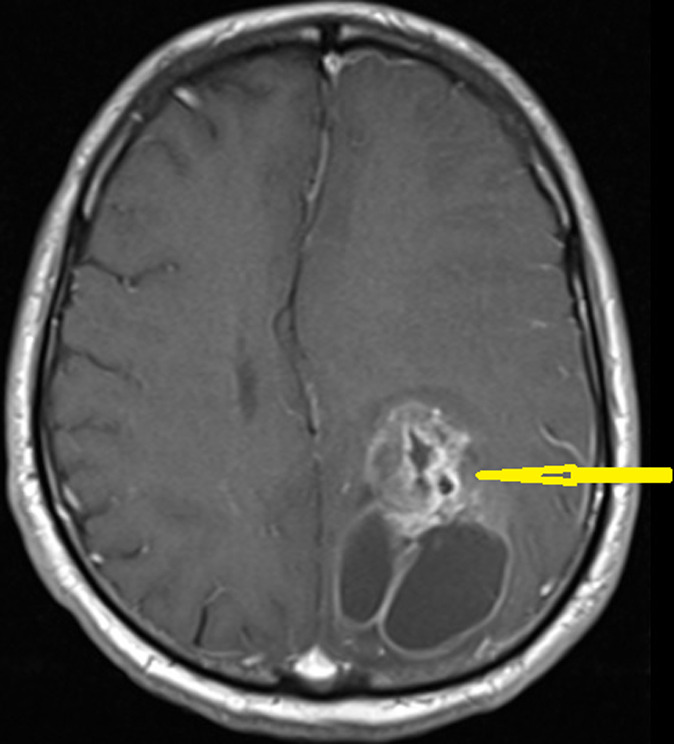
brain magnetic resonance imaging (MRI) shows brain metastasis (arrow)
